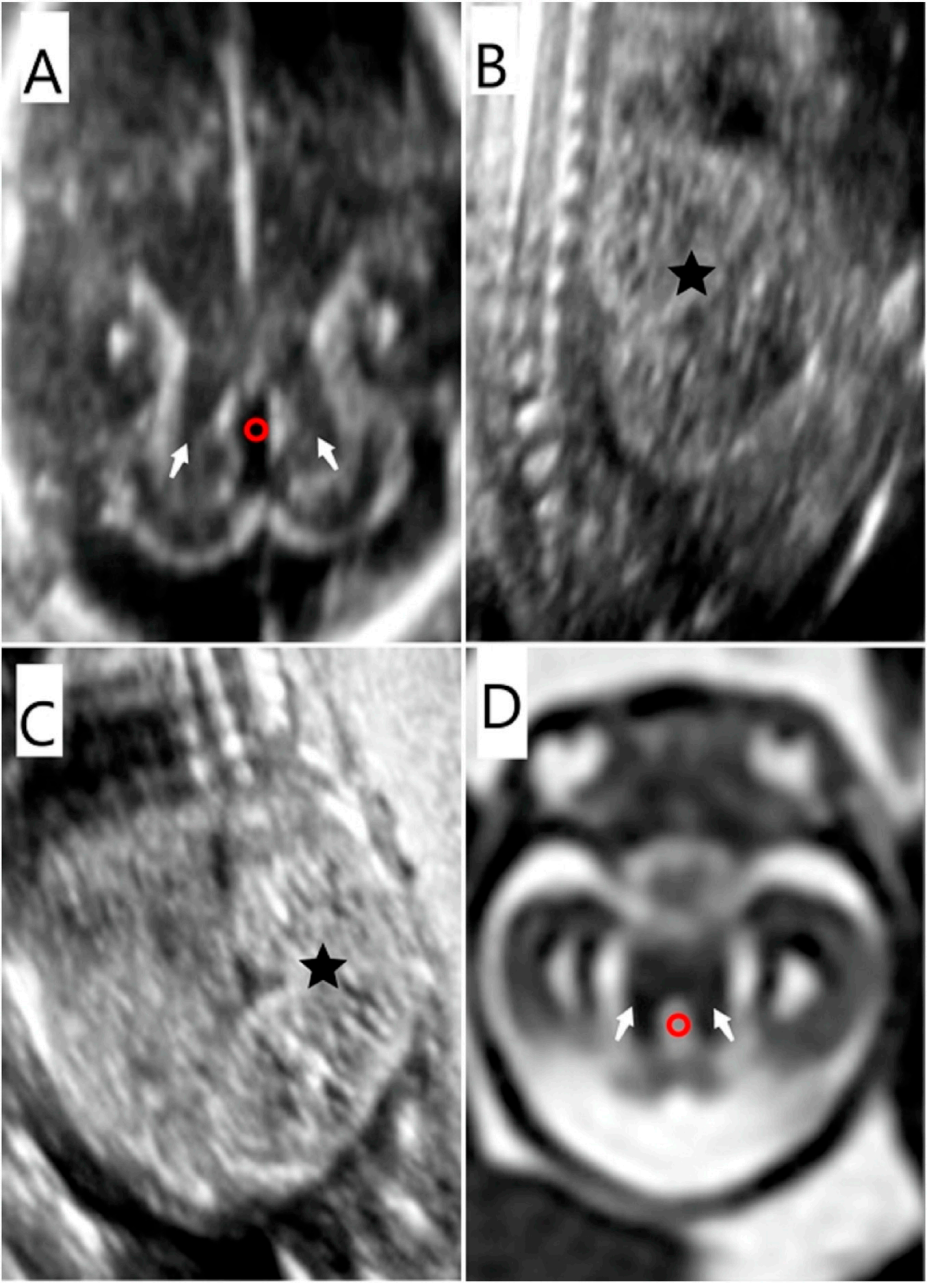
Prenatal imaging features of the fetus in case 7. (A) (D) MTS (white arrow) was detected by US and MRI. The fourth ventricle presented as “elongated” (red circle) on US and MRI. (B) (C) Bilateral polycystic kidneys (★) were identified by ultrasound.
